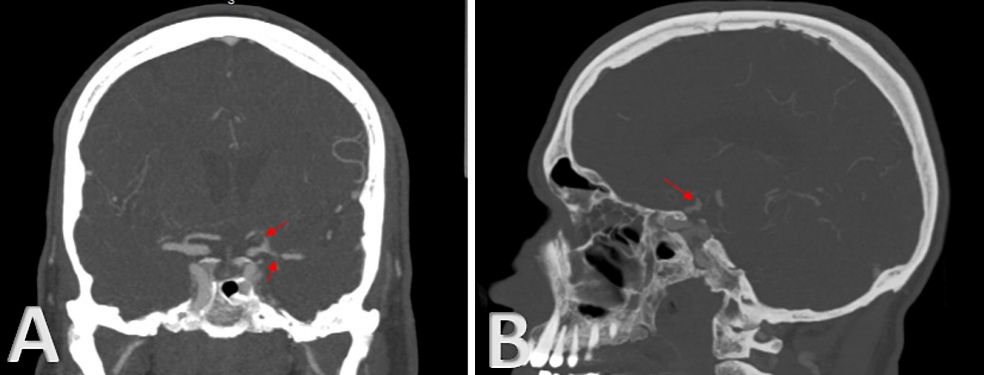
(A) Coronal view of CTA head demonstrates severe stenosis in left A1 and M1 (red arrows). (B) Sagittal view of CTA head demonstrates moderate stenosis in the left ICA terminus (red arrow). CTA: computed tomography angiography; ICA: internal carotid artery
